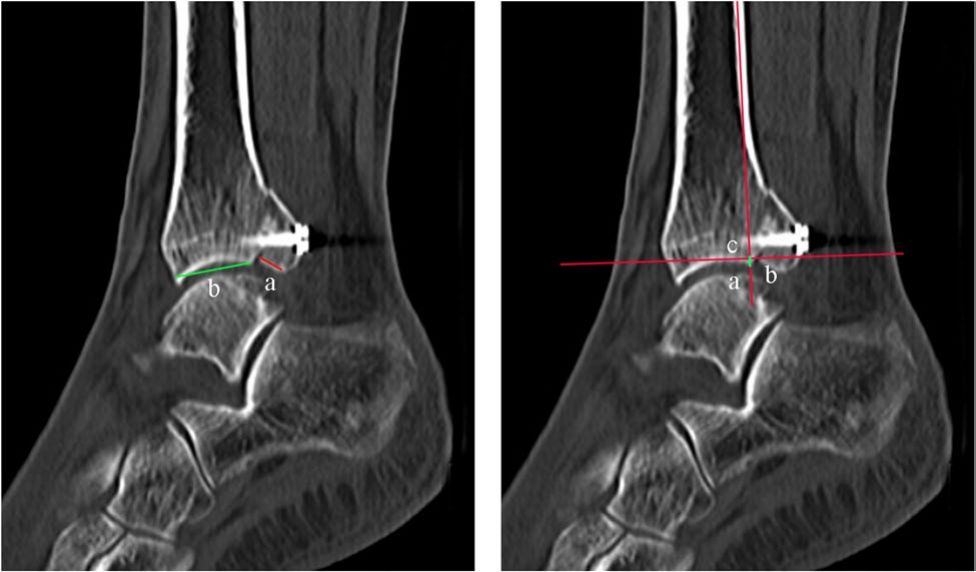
CT radiographic measurement of patirents. A Percentage of posterior malleolar fractures on the articular surface: a/(a+ b) × 100% (a. length of the articular surface of the posterior malleolar fragment; b. length of the articular surface of distal tibia). B Posterior malleolar fracture step-off: ac distance (a. fracture point of the articular surface of distal tibia; b. fracture point of the articular surface of the posterior malleolar fragment; c. intersection between the long axis passing point a and being parallel to tibia and the long axis passing point b and being perpendicular to tibia)
